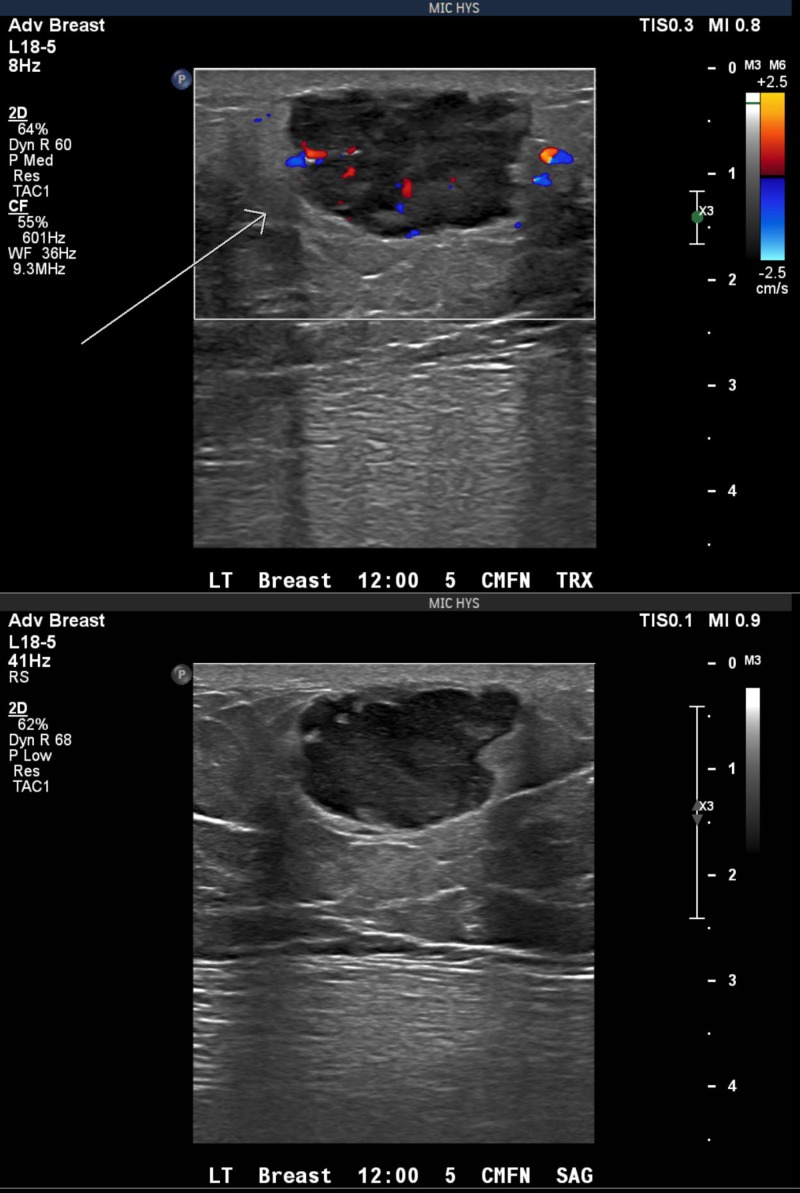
Ultrasound of the left breast showing increased vascularity
